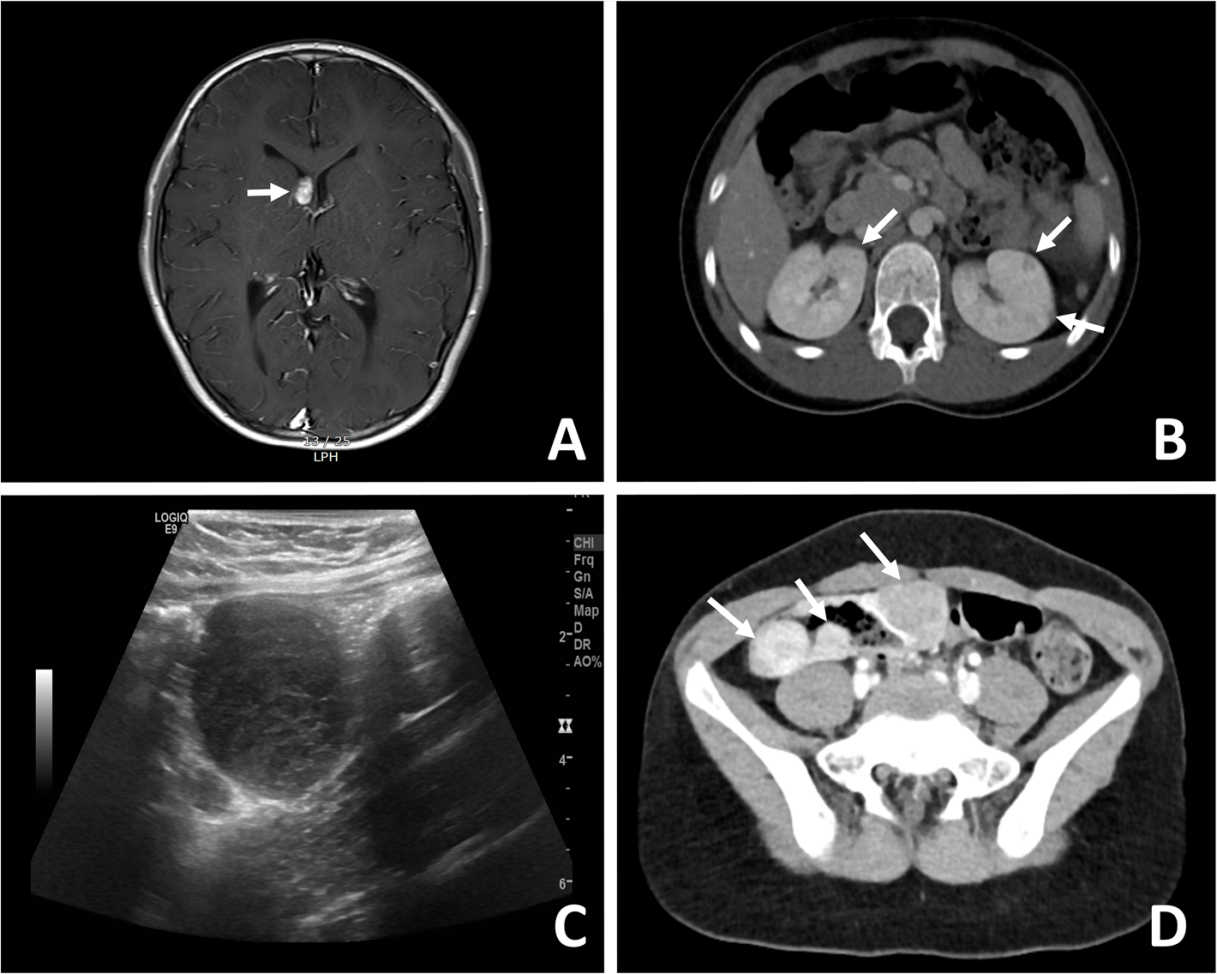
A Brain MRI showing an ovoid, enhancing mass at the right lateral ventricle (arrow). B Abdominal CT scan showing multiple low-attenuated foci in both kidneys (arrows). C Ultrasonography findings showing multiple low echoic nodules along the bowel wall. D Abdominal CT scan showing multiple enhancing masses along the ascending colon wall (arrows). MRI, magnetic resonance imaging; CT, computed tomography
